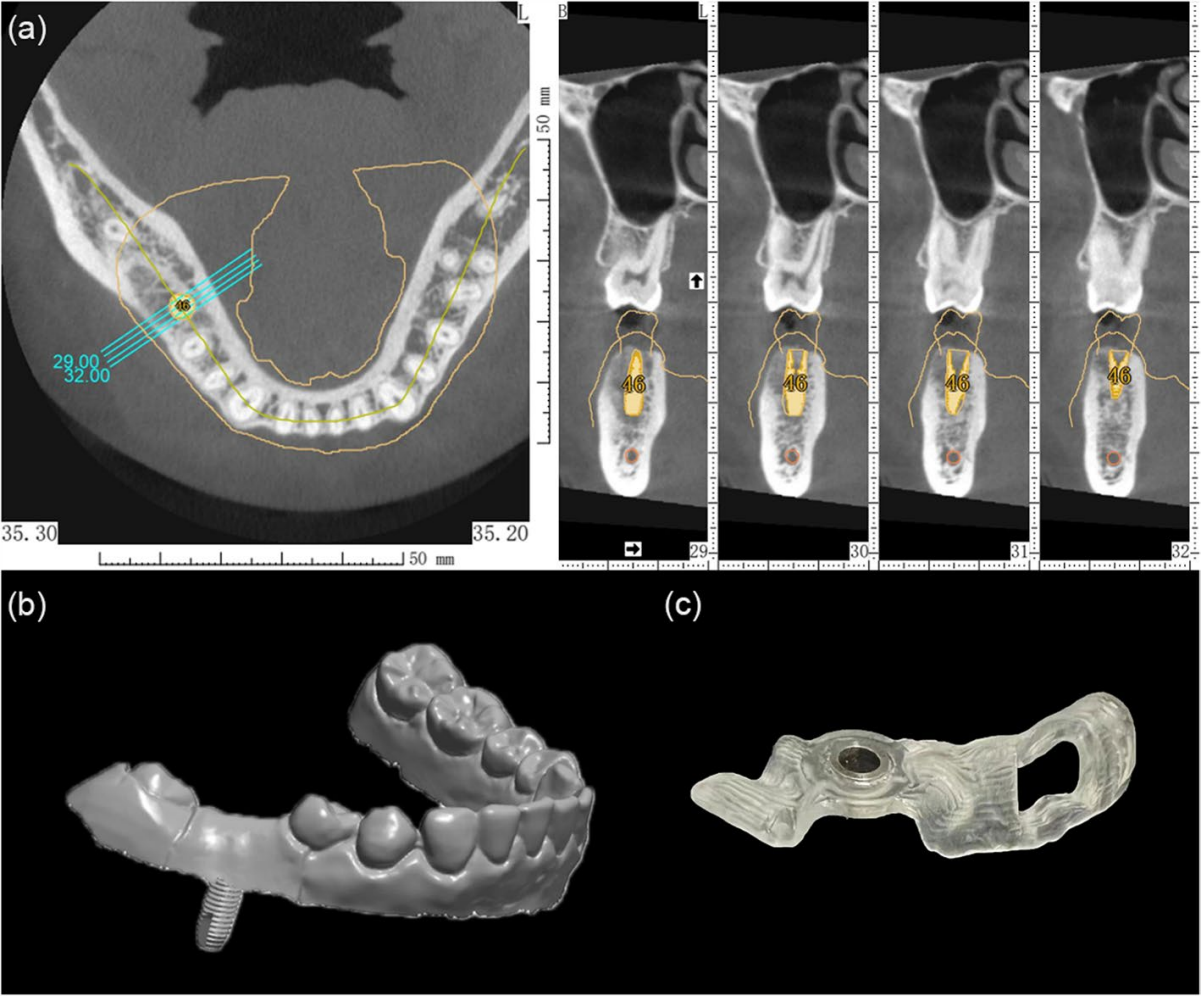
Presurgical digital design and fabrication of the surgical template. a Prosthetic and biological-driven digital design of implant position based on the superimposed CBCT data and optical scanning data; b Generation of a STL model based on presurgical design; c Fabrication of the CAD/CAM surgical guide
